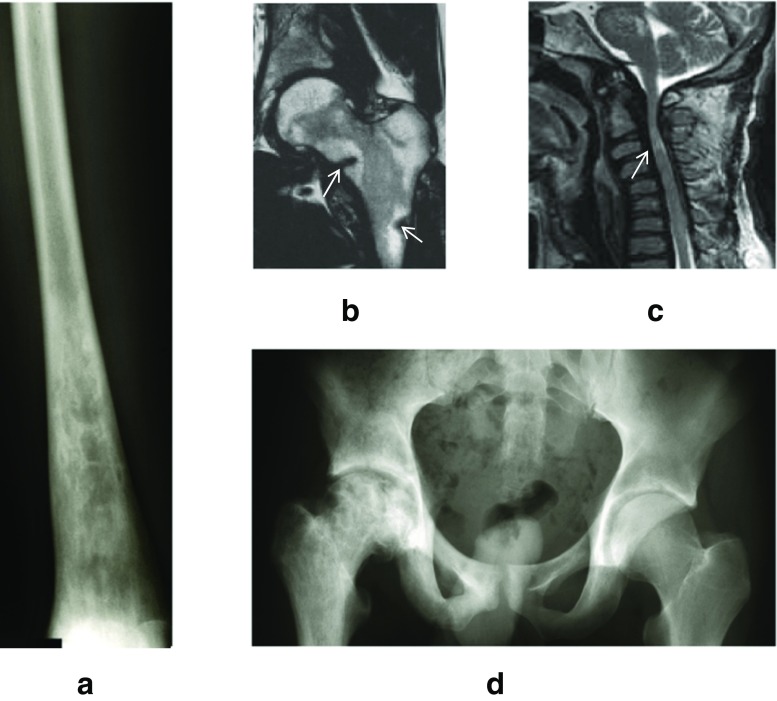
Examples of typical skeletal abnormalities in patients with inborn errors of metabolism. a. Erlenmeyer flask deformity in the distal femur of a patient with type 1 Gaucher disease (plain radiograph). b. Fragility fractures (arrows) in the femoral neck and diaphysis of a patient with X-linked hypophosphatemic rachitis (T2-MRI). c. Myelopathy (arrow) due to narrowing of the spinal canal in MPS-I. Note the platyspondylia (flattened vertebral bodies) as part of the dysostosis multiplex (T2-MRI). d. Avascular necrosis on the right side, normal femoral head on the left in a patient with Gaucher disease (plain radiograph)
